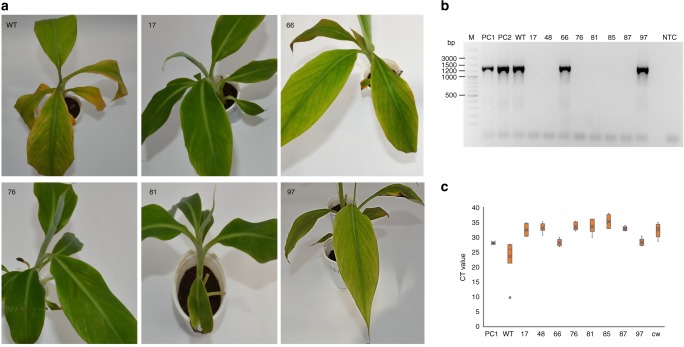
Evaluation of genome-edited and wild type non-edited control plants of Gonja Manjaya for induction of BSV symptoms under water stress conditions. Two-month-old plants were subjected to water stress for 2 weeks. Disease symptoms as chlorosis or yellow streaks were recorded at the end of the stress period and pictures were taken. a Pictures of asymptomatic genome-edited plants (17, 76, and 81), symptomatic-edited plants (66 and 97), and wild type control plants (WT). b PCR diagnostic to detect activation of episomal BSOLV in genome edited and control plants under water stress conditions. c qPCR analysis to detect episomal BSOLV in genome edited and control plants under water stress conditions. PC1‒2, symptomatic plant of Agbagba from field as positive control; WT, wild type non-edited control Gonja Manjaya plant under stress conditions; 17, 66, 76, 81, 97, edited plants under stress conditions; CW, asymptomatic in vitro plantlet of Cavendish Williams as negative control; NTC no template control. For PCR and qPCR, leaf samples from three symptomatic wild type non-edited control plants (WT) of Gonja Manjaya were pooled for DNA extraction. Similarly, the leaves from three replicates for symptomatic and asymptomatic-edited events were pooled for PCR and qPCR. CT values were presented as means and standard error of six technical replicates from two independent experiments
